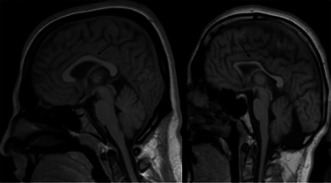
FIGURE 1 Sagittal T1‐weighted brain MRI from two SPG11 patients showing marked thinning of the corpus callosum (TCC), as representative examples of the most common radiological finding in SPG11 (arrows).
